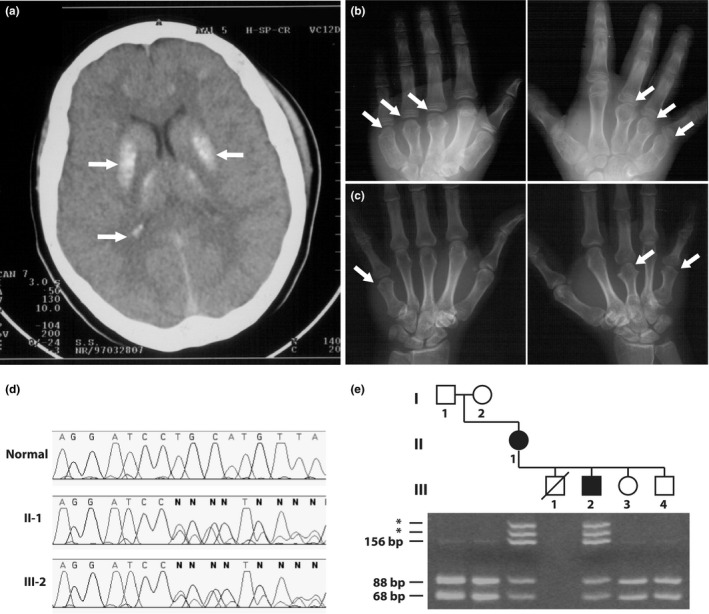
(a) Head computed tomography (CT) scan of the affected child showing calcification of basal ganglia and of other dispersed regions of the brain (arrows). (b) Radiograph showing shortening bilaterally of the 3rd, 4th and 5th metacarpals (arrows) in the affected child, typical of Albright's osteodystrophy. (c) Shortening of the left 5th, and right 3rd and 5th metacarpals in the mother who has pseudopseudohypoparathyroidism (PPHP). (d) DNA sequence analysis of both mother (II‐1) and child (III‐2) revealed a frameshift that resulted from a heterozygous 2‐base pair (bp) deletion at codon 63 (c.188_189delTG). The mutation nomenclature was based on the *GNAS* cDNA reference sequence (GenBank Accession number NM_000516.4). (e) This 2‐bp deletion resulted in the loss of an *Nsp*I restriction enzyme site in the mutant sequence, and this facilitated the screening of the mutation in other family members. Electrophoresis of *Nsp*I digested PCR fragments on an 8% polyacrylamide gel showed that one product of 156 bp was obtained from the mutant sequence (additional heteroduplex products are indicated by asterisks), but two products of 88 and 68 bp were obtained from the wild‐type normal sequence. The affected individuals are heterozygous, and the unaffected are homozygous for the wild‐type allele. Individuals are represented as males (squares), females (circles), unaffected (open symbol), affected (filled symbol) and deceased (oblique line through symbol). Individual III‐1 was reported to share a similar phenotype as III‐2 by his relatives.
